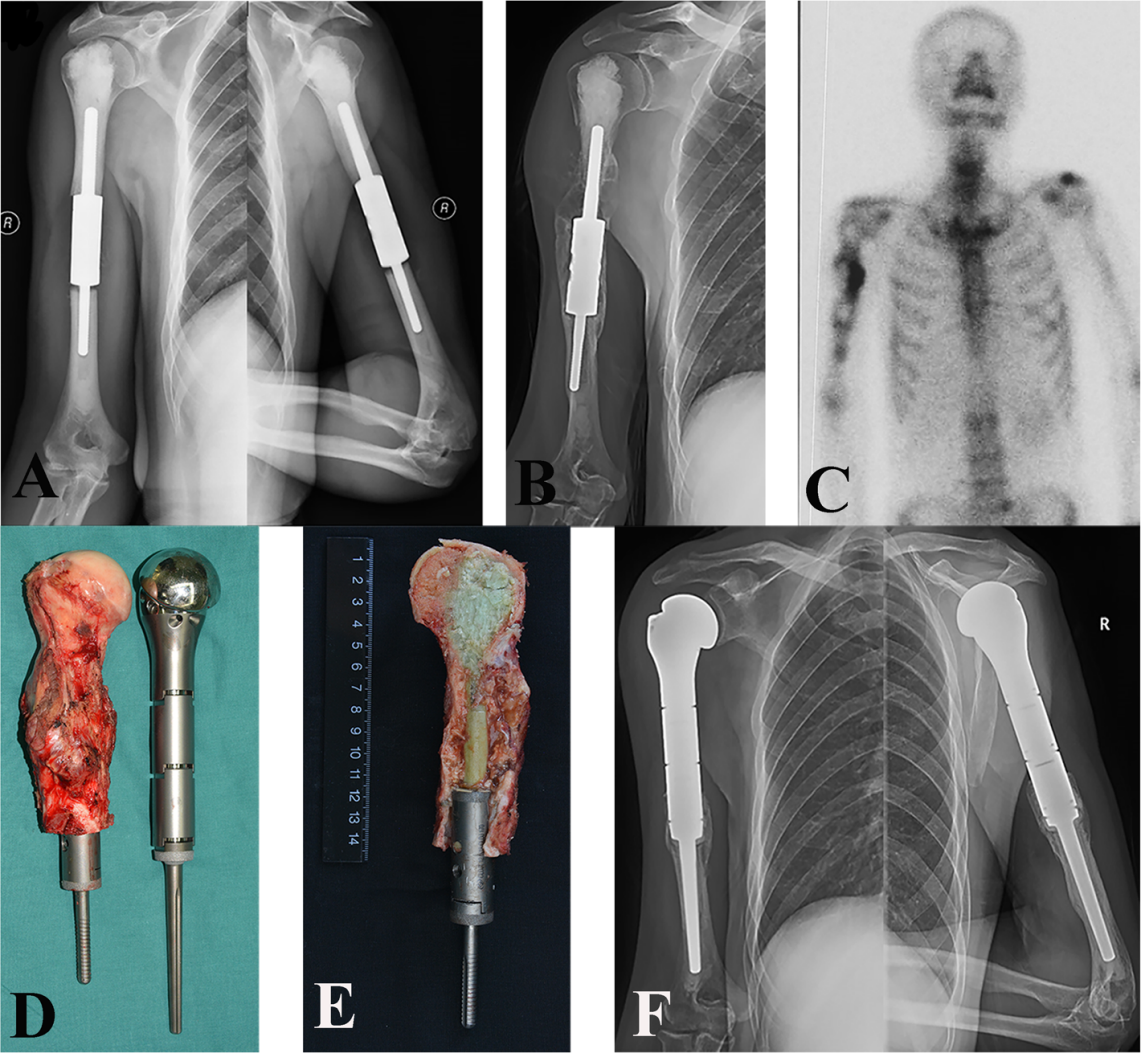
a Postoperative radiographs of a 60-year-old man who underwent segmental resection and reconstruction by using a cemented custom-made segmental prosthesis. b, c The X-ray and bone scintigraphy scan taken 48 months show local relapse proximal to the humerus and loosening of the distal stem. d, e The intraoperative pictures showed a lesion at the proximal humerus, and then, revision with a modular proximal humeral prosthesis was performed. f The postoperative image shows that the position of the prosthesis is satisfactory
